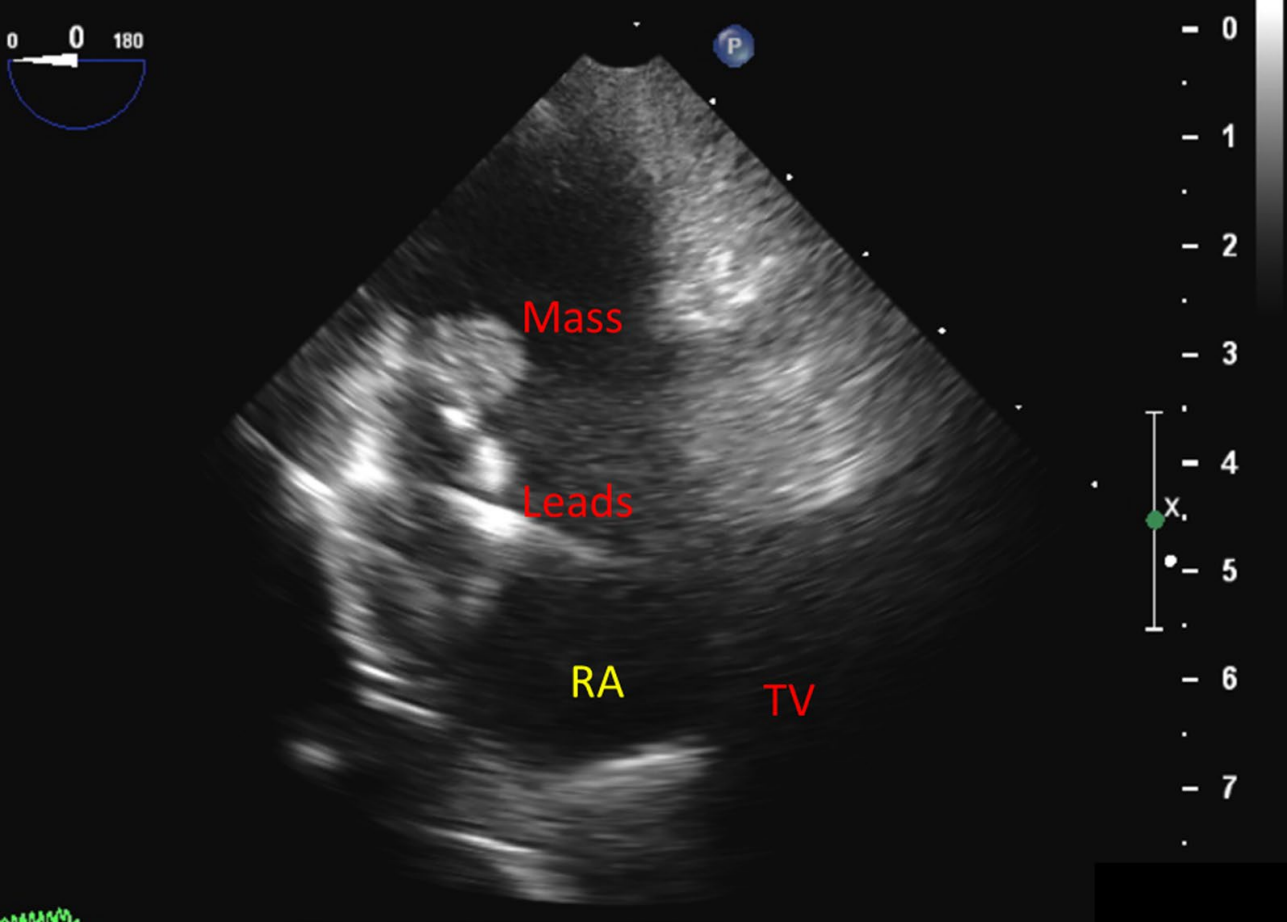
Transesophageal echocardiogram at mid esophageal level showing mass in the right atrium attached to the lead
